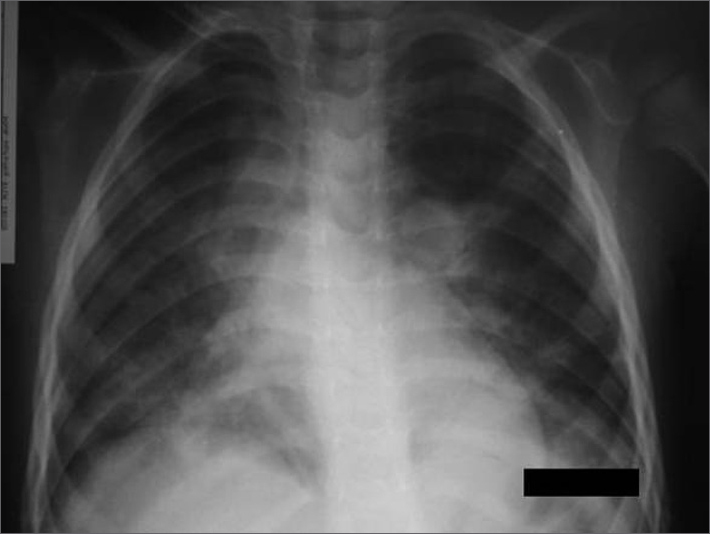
Chest X-Ray during respiratory dysfunction, showing the bilateral interstitial infiltrate.
